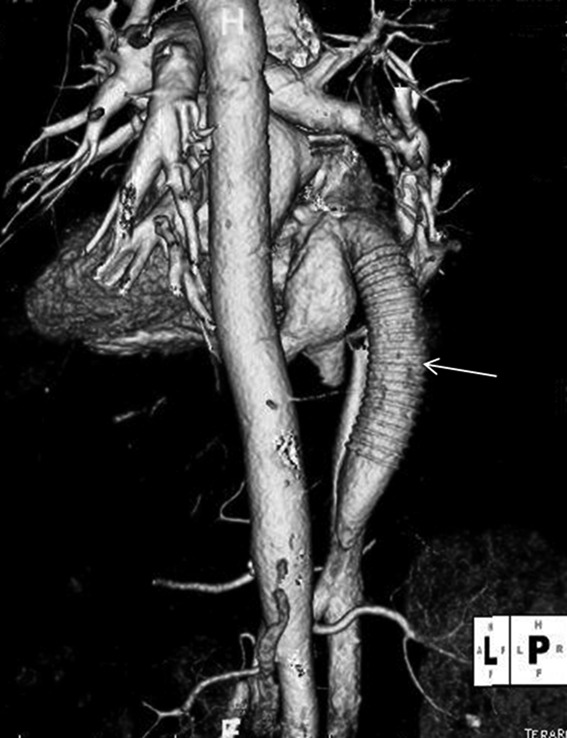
Postoperative reconstructed CT image shows the ringed PTFE graft (arrow) from suprarenal IVS to right atrium with good blood flow.
